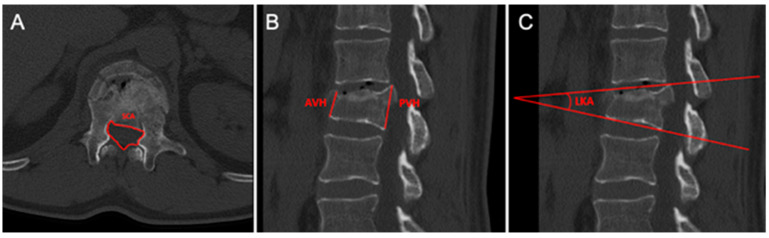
CT general radiological parameters. (A) SCA (spinal canal area). (B) AVH (anterior vertebral height) and PVH (posterior vertebral height). (C) LKA (local kyphotic angle).
